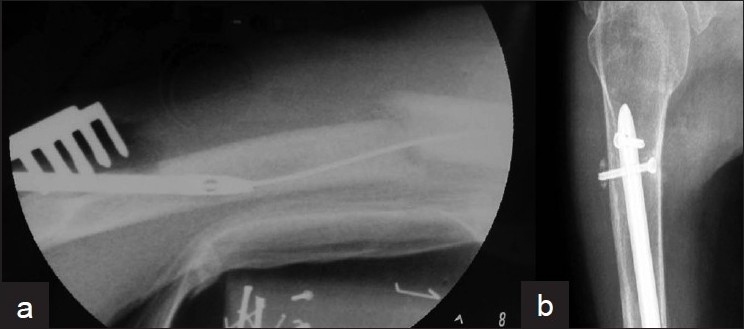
(a) Lateral radiograph of lower arm depicting that the danger of a supracondylar fracture during retrograde nailing is substantial, due to entry hole made for nail insertion. (b) X-ray of the upper arm (lateral view) depicting that locking proximally a retrograde nail could be facilitated if performed below the surgical neck of the humeral head
